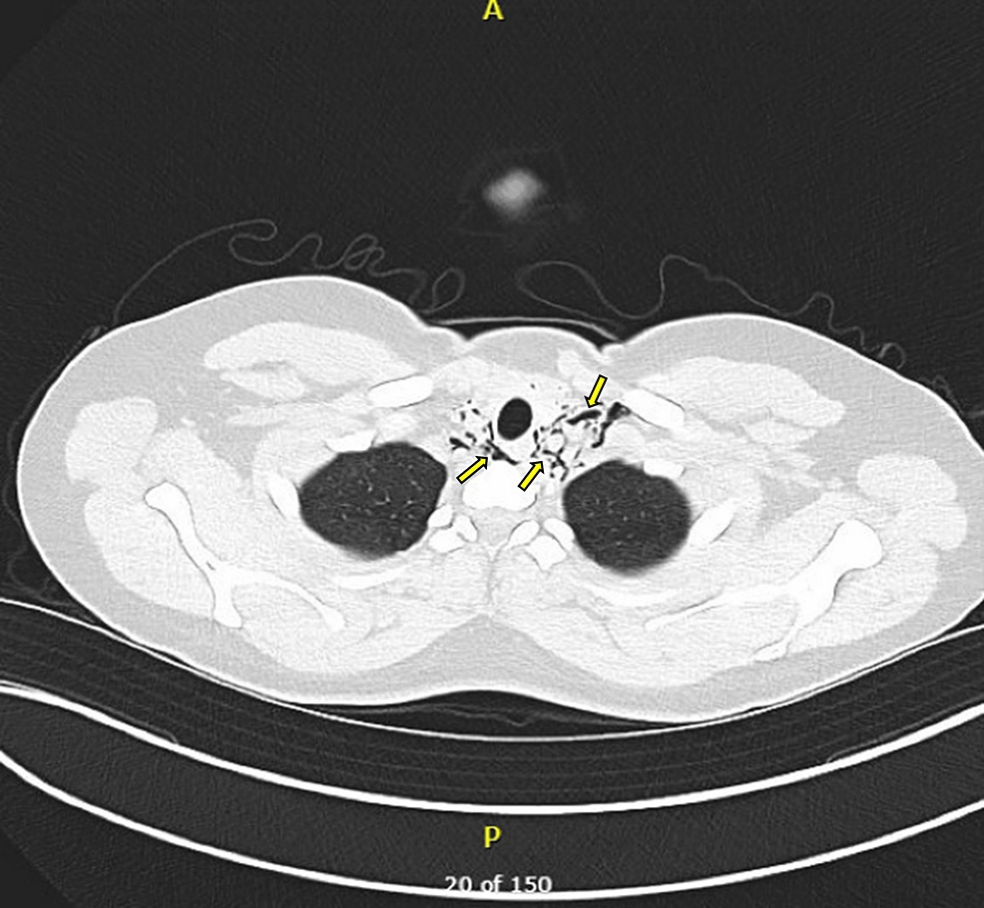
CT neck of subcutaneous emphysema extending into the neck Axial CT neck with soft-tissue window demonstrating subcutaneous emphysema extending into the neck. Arrows denote air pockets surrounding the carotid and paratracheal neck space and subcutaneous tissue CT: computed tomography
